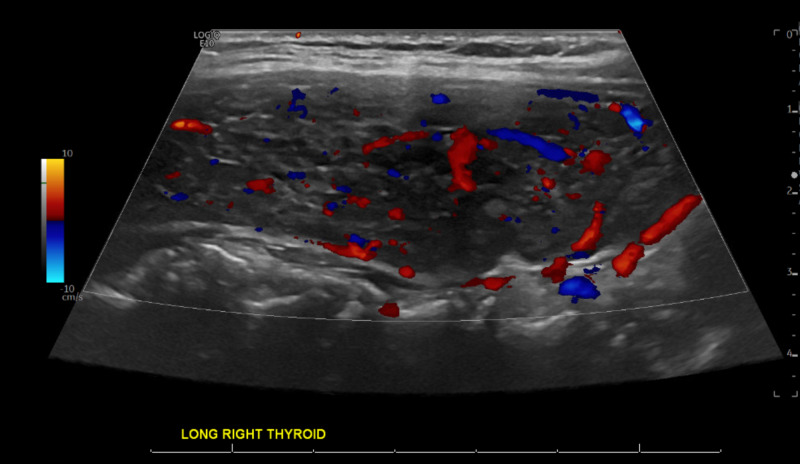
Thyroid doppler ultrasonography, longitudinal view: increased asymmetric vascularity in the right thyroid lobe indicative of Hashimoto thyroiditis
